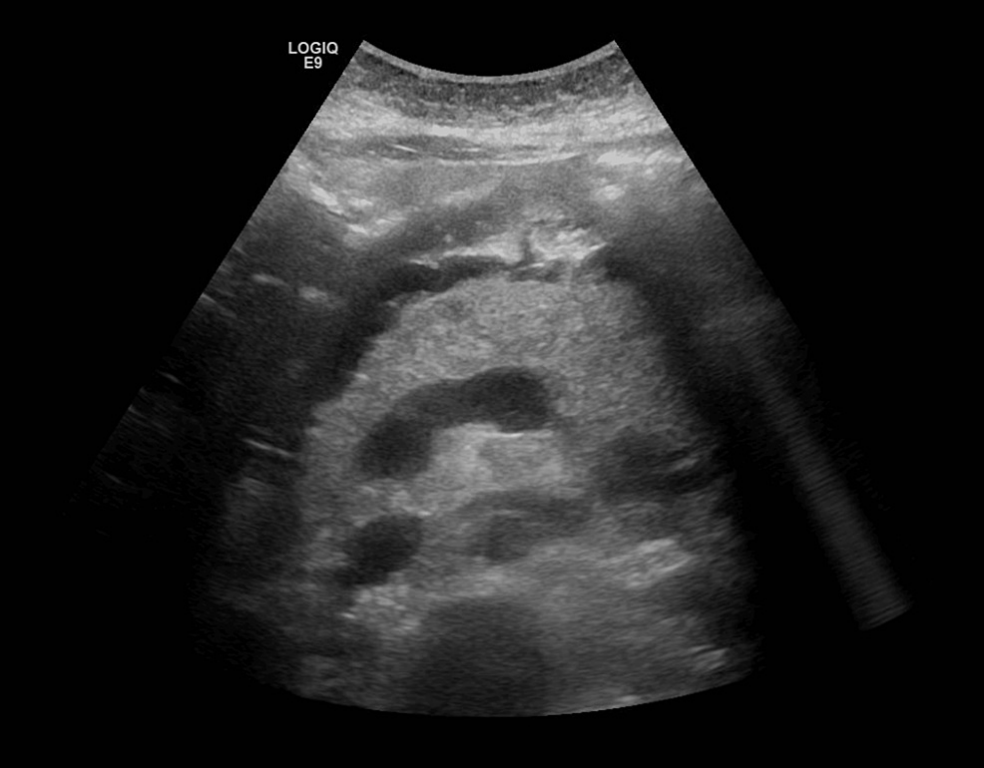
Ultrasound abdomen showing diffusely enlarged pancreas more at the tail with an irregular surface and surrounding peri-pancreatic free fluid suggestive of acute pancreatitis
